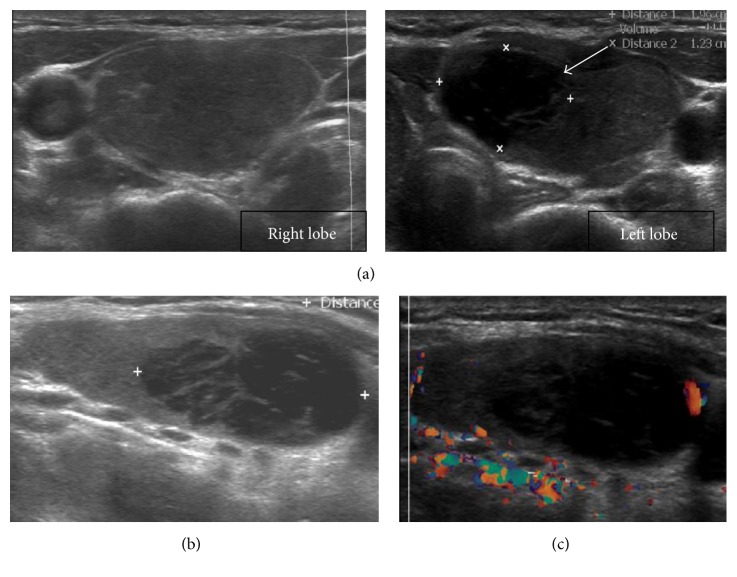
(a) Thyroid ultrasound shows a markedly hypoechoic area (arrow), with interspersed linear echogenic strands pattern in transverse view and (b) longitudinal view. (c) Color Doppler image shows a left thyroid nodule with peripheral increased blood flows in longitudinal view during a first visit.
